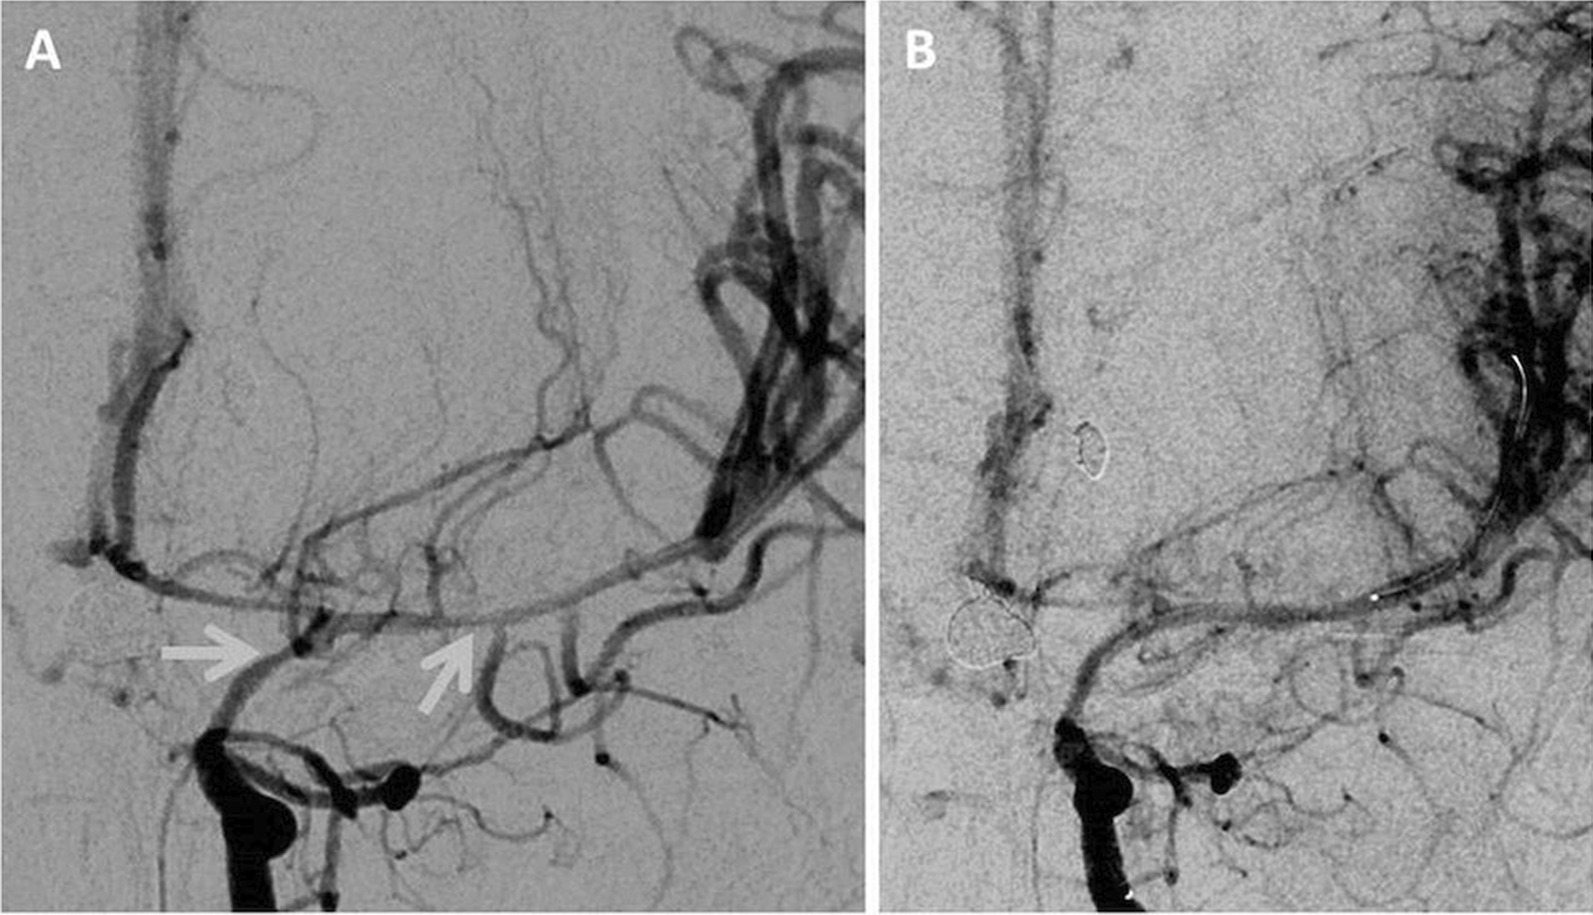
A Anteroposterior view of a cerebral angiogram study showing radiographic evidence for vasospasm, with narrowing identified at the supraclinoid portion of the left internal cerebral artery and along the left middle cerebral artery (shown by gray arrows). B Radiographic improvement following injection of intra-arterial milrinone and balloon angioplasty
